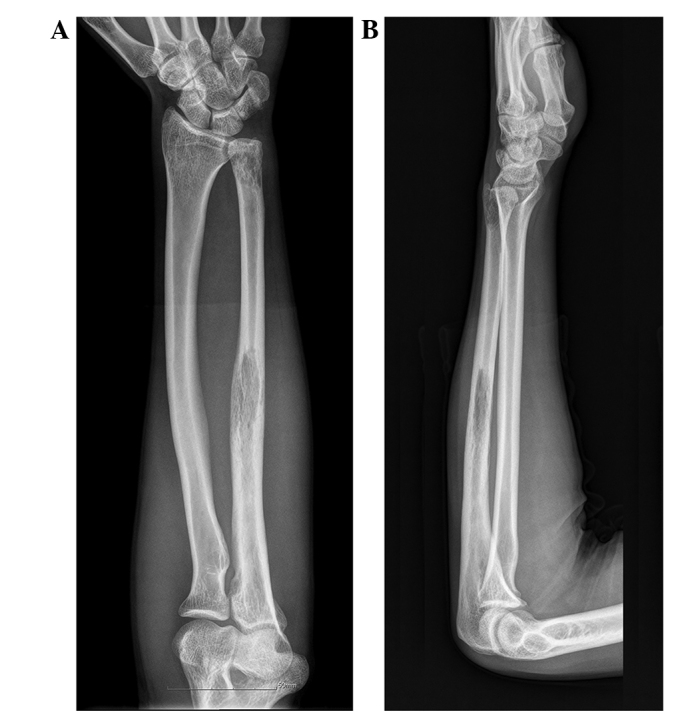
(A) Anteroposterior and (B) lateral plain radiographs showing an osteolytic lesion with cortical destruction in the proximal, middle and distal ulna.
